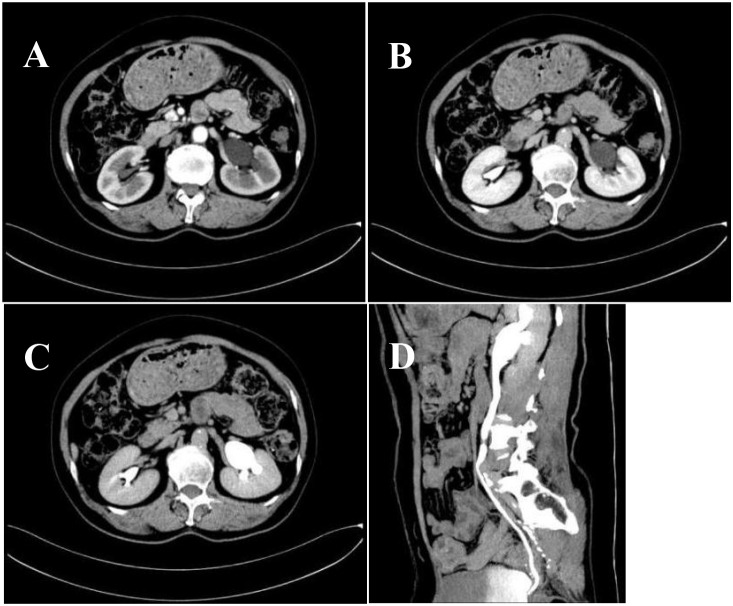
Contrast-Enhanced CT: (A) Arterial phase; (B) Venous phase; (C) Excretory phase; (D). The middle segment of the left ureter is not visible in the coronal position.
